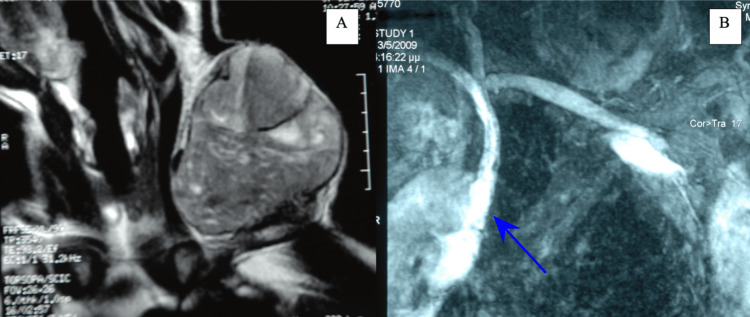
MRI and MRA images of the mass A: MRI shows a well-defined, multi-lobulated mass with heterogeneous signal intensity due to tissue mineralization. B: MRA coronal view shows peripheral/septal enhancement.
